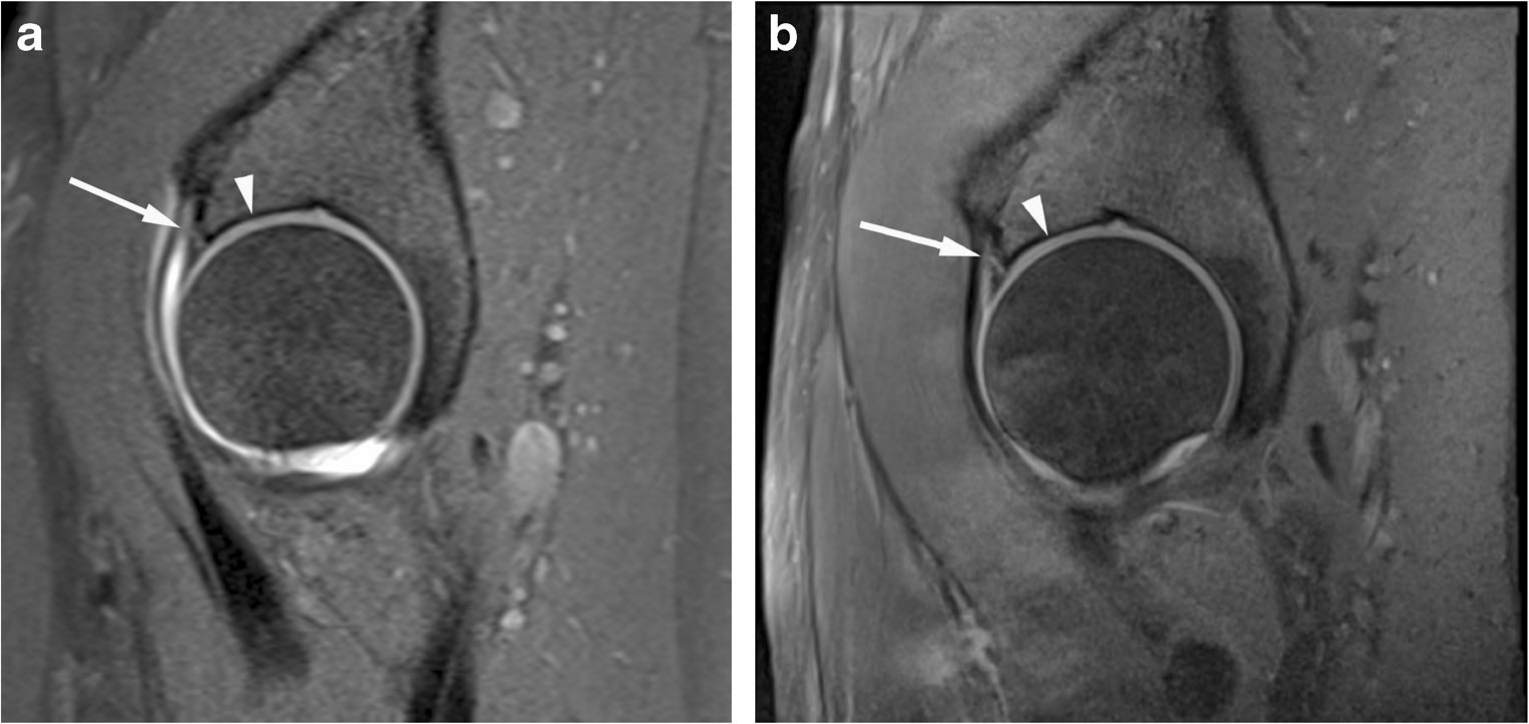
Acetabular labrum complete tear in a 27-year-old confirmed at surgery. Sagittal (a) T1 FS 1.5T MRA and (b) PD FS 3T MR images show complete basal labral tear (arrow) and normal articular cartilage (arrowhead)
